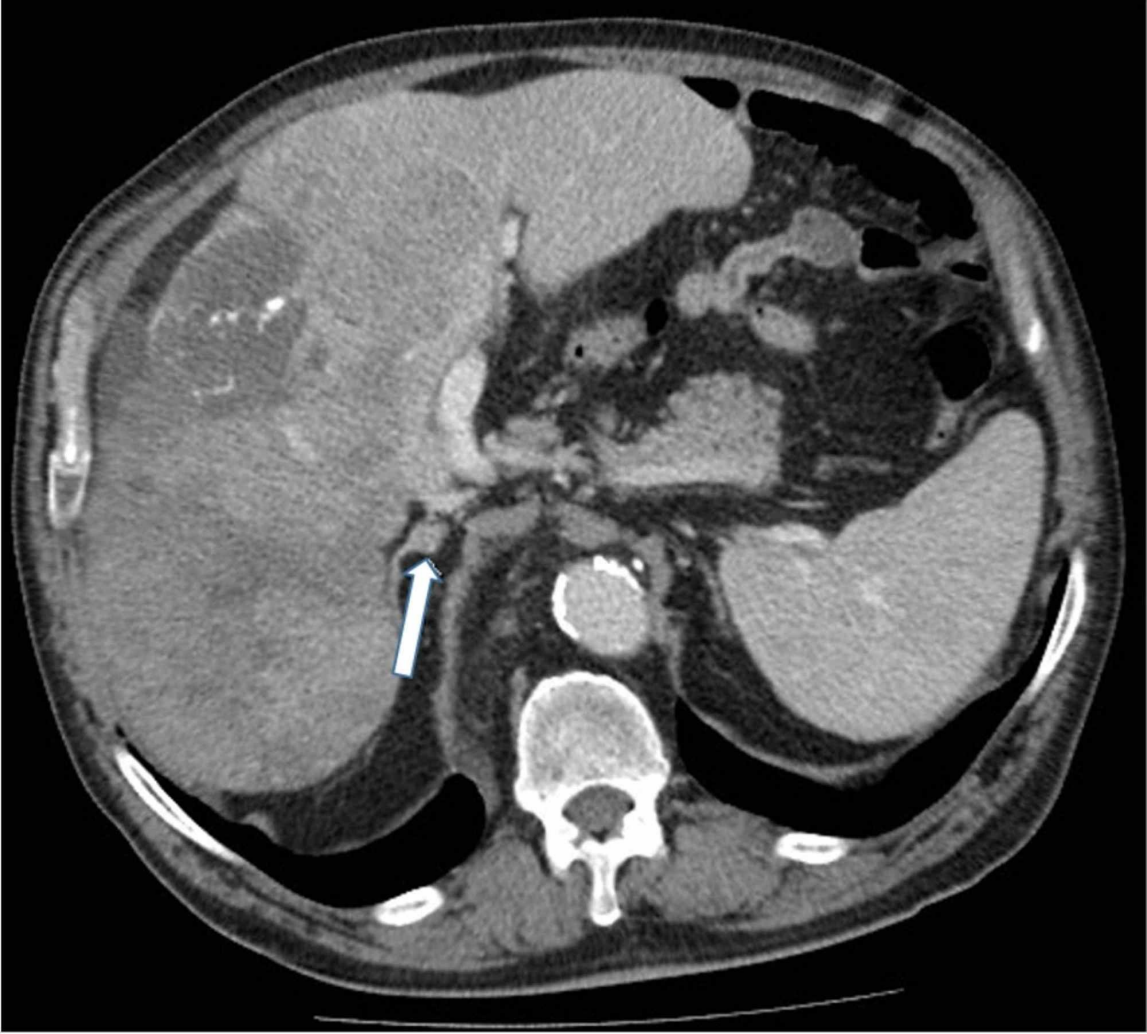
Mass in the right adrenal gland. A small mass (arrow) in the right adrenal gland was visible in the axial CT.
